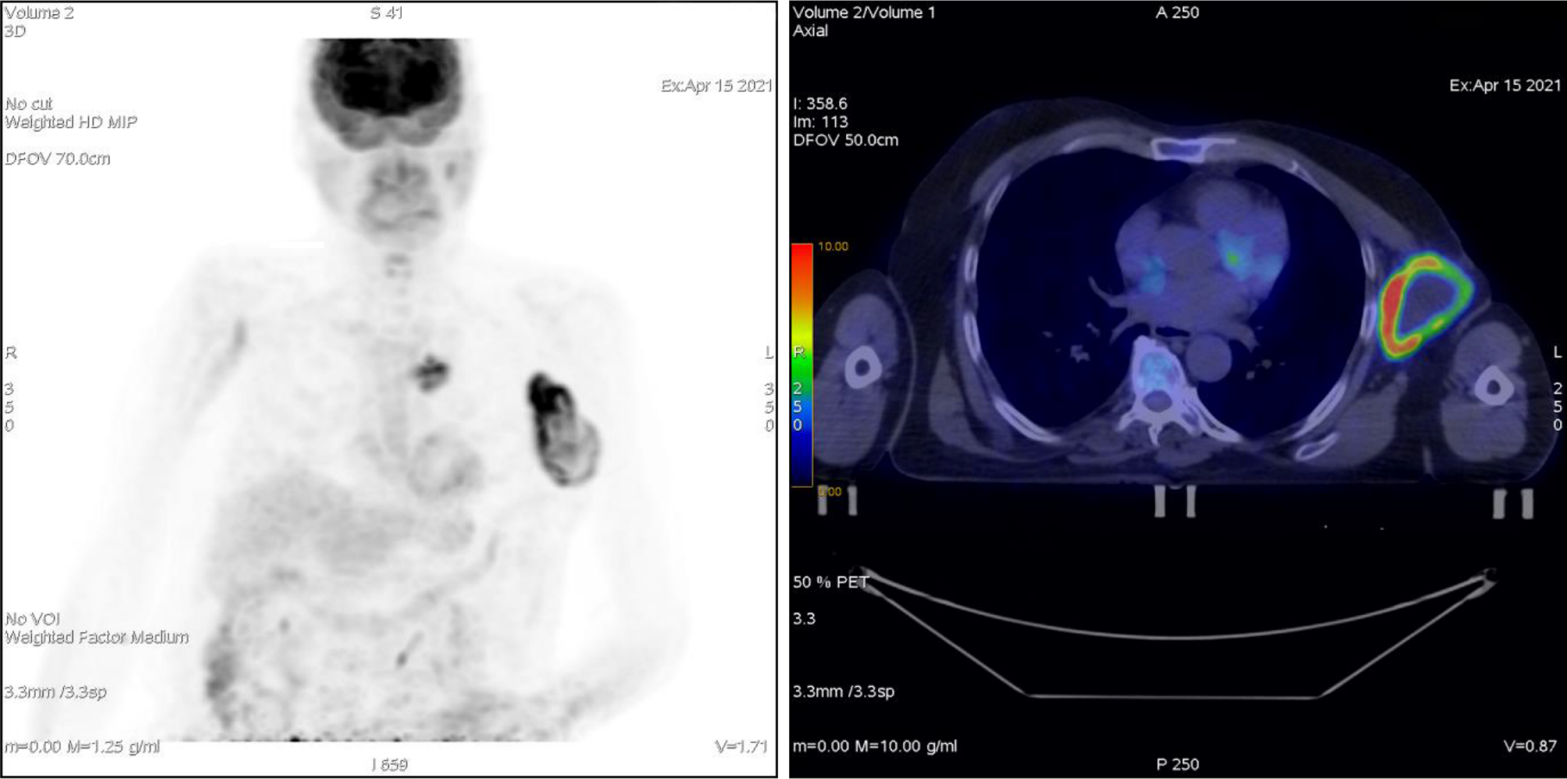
Patient ^18^F-FDG PET at presentation.
